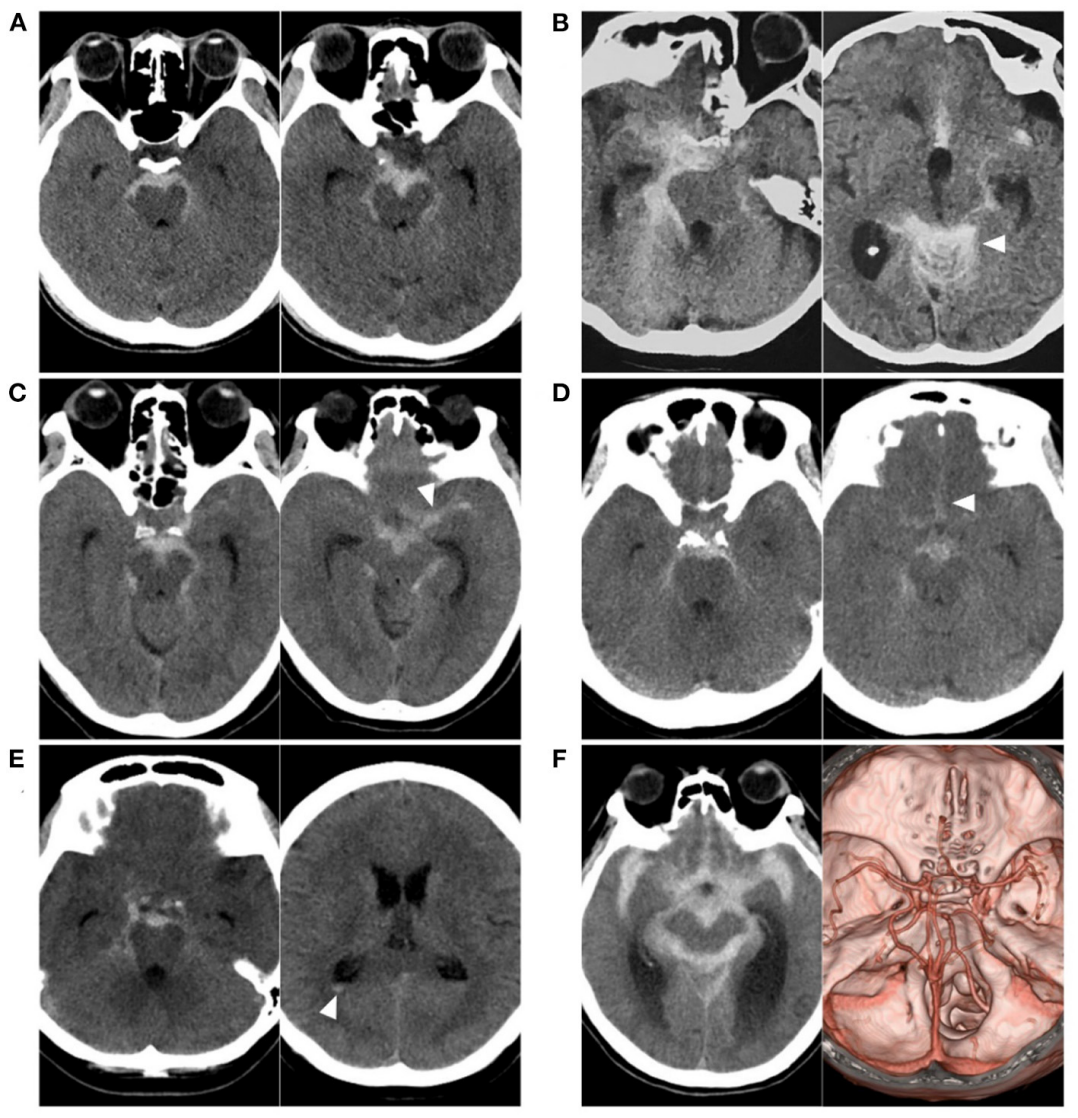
Various SAH with negative angiography. (A) CT showing a PNSAH only with focal pre-pontine hemorrhage. (B) CT showing a PNSAH with involvement of quadrigeminal cistern (arrowhead). (C) CT showing a PNSAH extended to the basal part of the Sylvian fissure (arrowhead). (D) CT showing a PNSAH extended to the posterior part of the interhemispheric fissure (arrowhead). (E) CT showing a PNSAH with intraventricular sedimented blood (arrowhead). (F) Left: CT showing a diffuse non-PNSAH; Right: the intracranial aneurysm was not found on CTA; the drainage pattern of the deep vein around vein of Galen was normal. CT, computed tomography; CTA, computed tomography angiography; PNSAH, perimesencephalic non-aneurysmal subarachnoid hemorrhage; SAH, subarachnoid hemorrhage.
